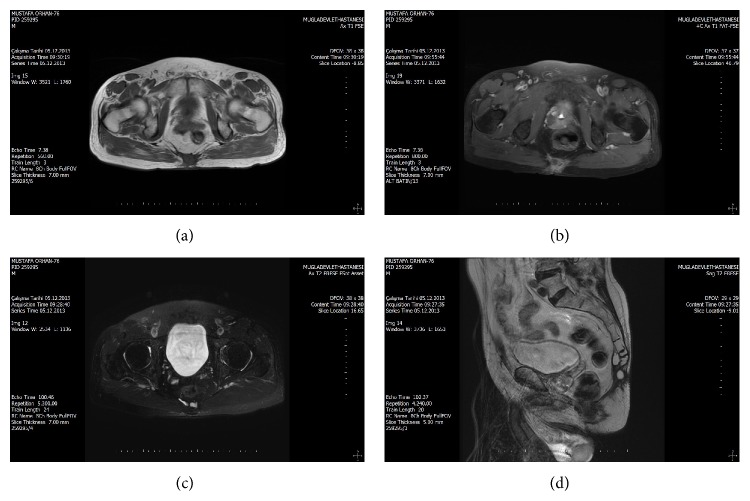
Magnetic resonance imaging. (a)–(d) A mass lesion was observed, which was separated with thin septations in the dimension of approximately 7 × 5.5 cm at* os coccyx* level, a part of which shines both in T1 and in T2, a part of which gives high signals in T1, and which had intense involvement of peripheral and septal heterogeneous contrast from postcontrast study.
